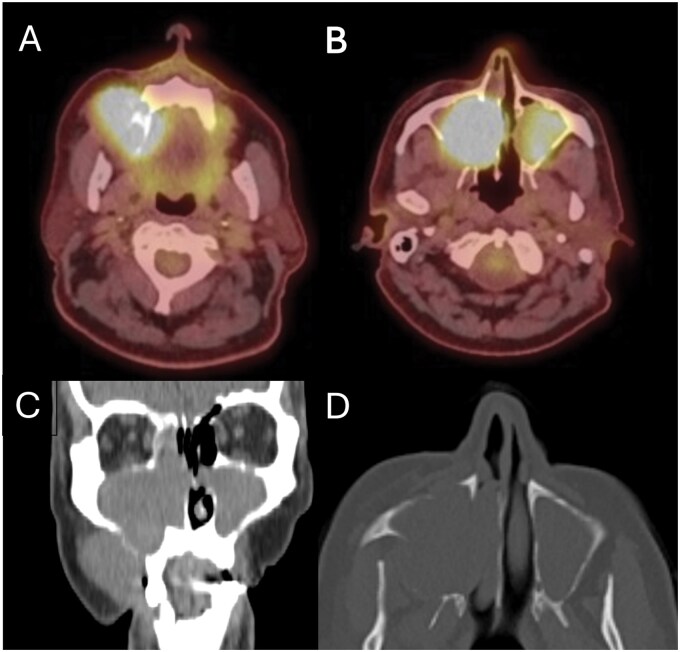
(A and B) A PET-CT study displaying hypermetabolic lesions in both maxillary antra. The degree of FDG-avidity is greater in the right maxillary antrum. (C and D) The non-contrast component of the study shows disease extending through the floor and posterolateral wall of the right maxillary sinus into the buccal sulcus and buccal space respectively.
